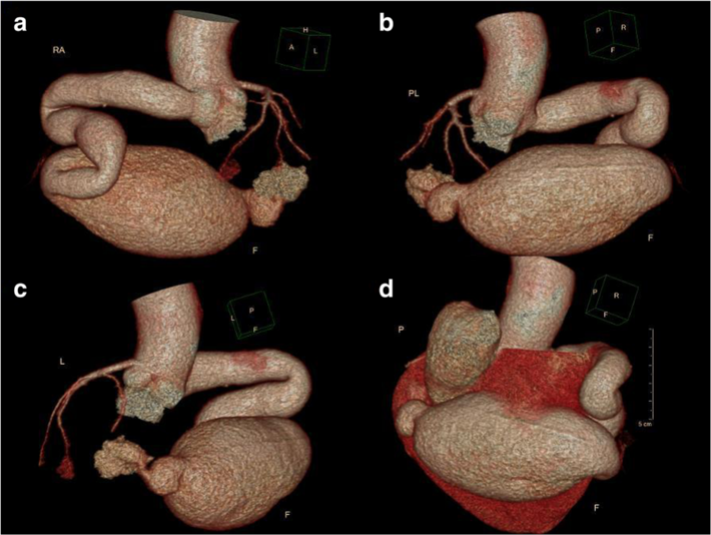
Coronary computed tomography angiography. Serpentine RCA and the giant saccular aneurysm located in the distal portions of the RCA, the diffusely dilated fistula drained into the LV: (a) left-anterior oblique view, (b) right-posterior oblique view, and (c) posteroanterior view. d The giant saccular aneurysm (up to 6.1 cm) surrounded the RV
